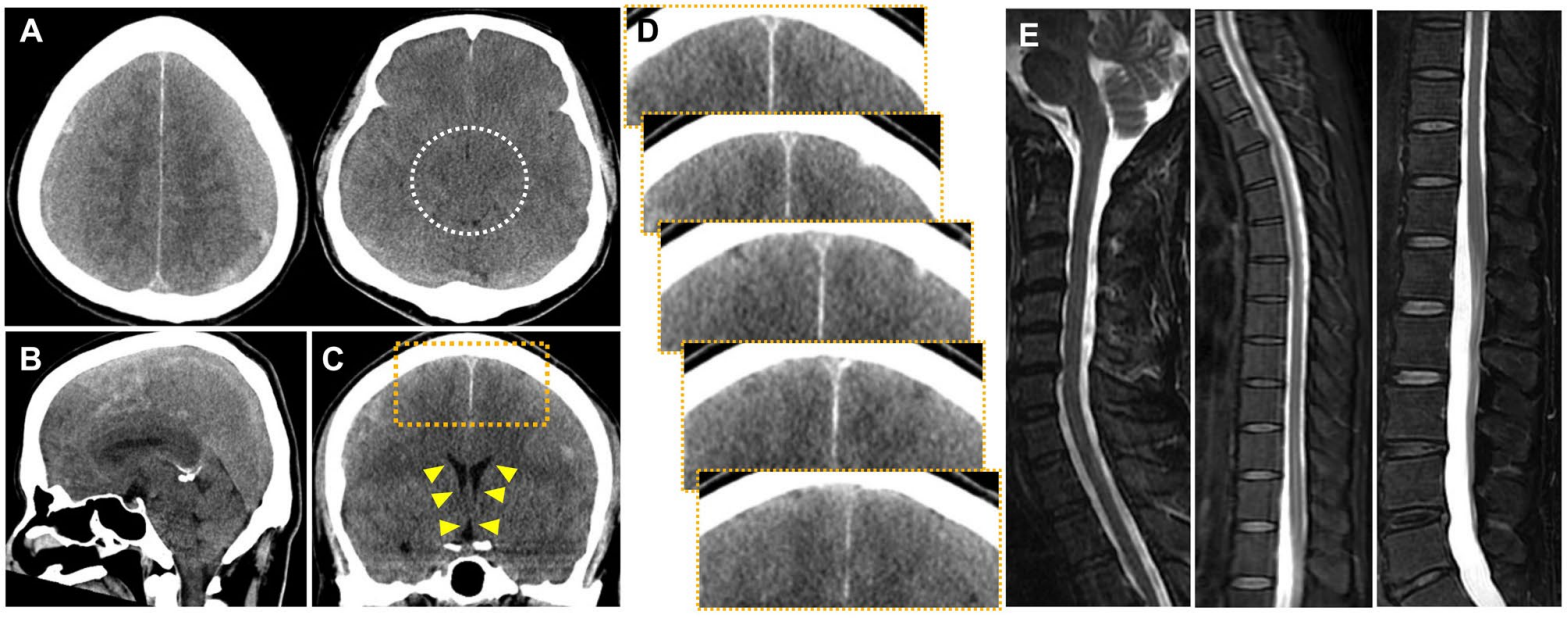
Initial CT findings of a case of bilateral CSDH without SIH (Case 20: male patient in his 40s, with two-month history of gradually worsening orthostatic headache). Head CT showing (A: axial) (Left) symmetric bilateral laminar and iso-hyperdense CSDH, which was categorized in Grade 1, (Right) obliteration of crural-and-ambient cisterns (white dotted circle), and (C: coronal) slit-like ventricles (yellow arrowheads). (B: sagittal) However, unlike SIH-induced case, cerebellar ptosis was not shown. Moreover, (D) cerebral sulci in the IHF and PSS were obliterated indicating possible non-SIH-related bilateral CSDH. (E) MRI indicated that there was lacking CSF leakage, diagnosing non-SIH-related CSDH
